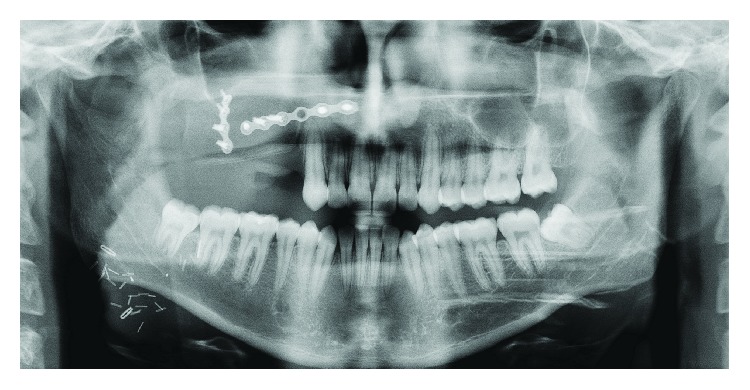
Panoramic X-ray after reconstruction by FRF.
